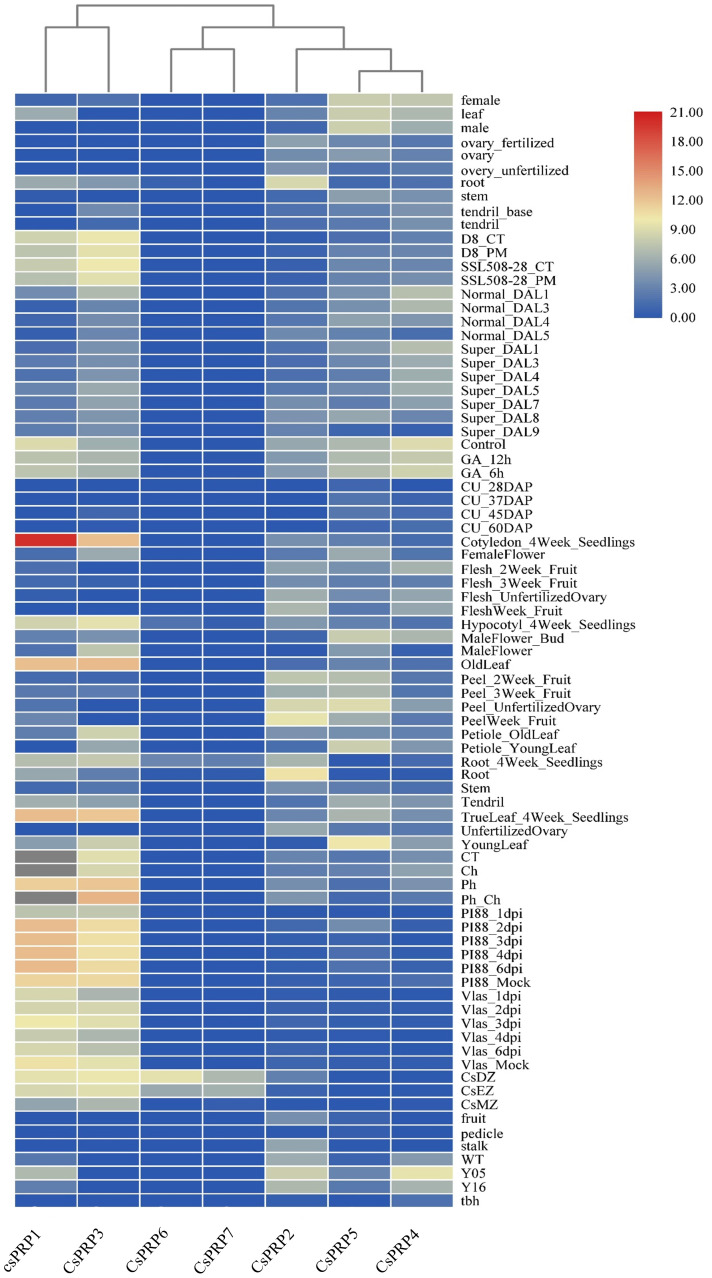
Expression pattern of cucumber PRP family. The sampling locations used for expression analysis in the figure include roots, leaves, seeds, ovaries, flowers, fruits, pedicels, and stems. The data presented is derived from RPKM that has been exponentially transformed and is represented by colors ranging from red to blue, with red indicating the highest expression and blue indicating the lowest. The abbreviations used in the figure are CT (control), DAP (day after pollination), DAL (ovary 4–5 days after flowering), Tbh (tiny branched hair mutant), Y05 (fruit thorns on 0.5 cm large fruits), Y16 (fruit thorns on 1.6 cm large fruits), WT (wild type cucumber), CsDZ (Root differentiation zone), CsEZ (Root elongation zone), CsMZ (Root meristematic zone), Ch (treated with chrysophanol), Ph (treated with formaldehyde), GA_ 12 hours (treated with gibberellin for 12 hours), and GA_ 6 hours (treated with gibberellin for 6 hours).
